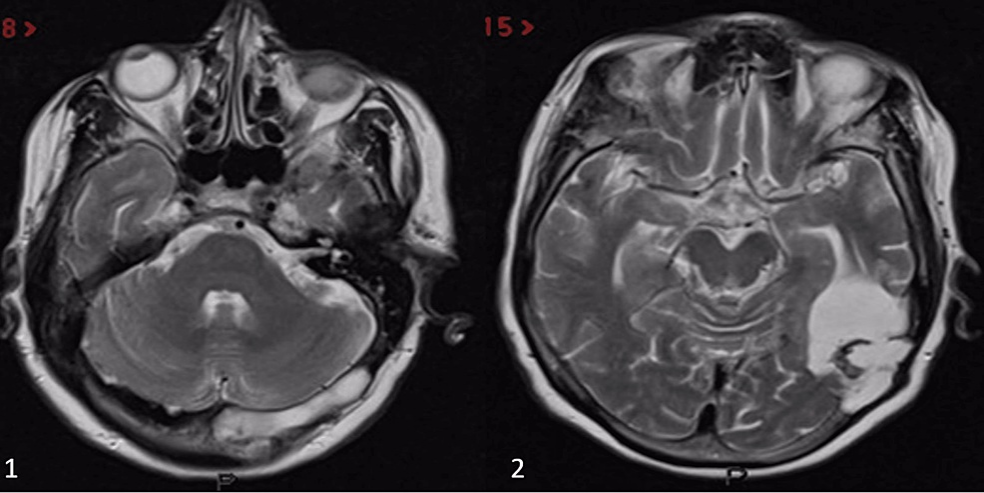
Preoperative axial T2-weighted MRI showing enlargement of the diploic distance extending towards the temporal bone. There was a CSF leakage at the opening of the mastoid cells. CSF: Cerebrospinal fluid
